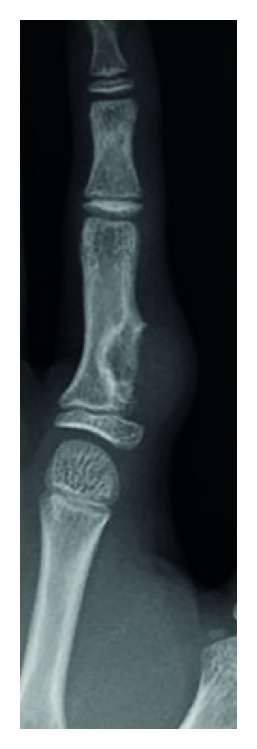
Anteroposterior radiograph reveals saucerization of the underlying cortex and a rim of sclerosis on the radial surface of the proximal phalanx.
